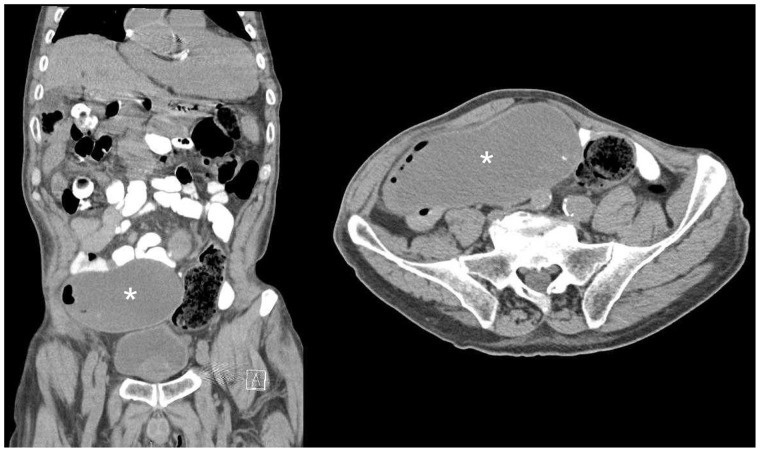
Computed tomography of the abdomen and pelvis, with oral contrast only in both the coronal and axial images. A white asterisk (*) in both views indicates the low-grade mucinous neoplasm of the appendix.
